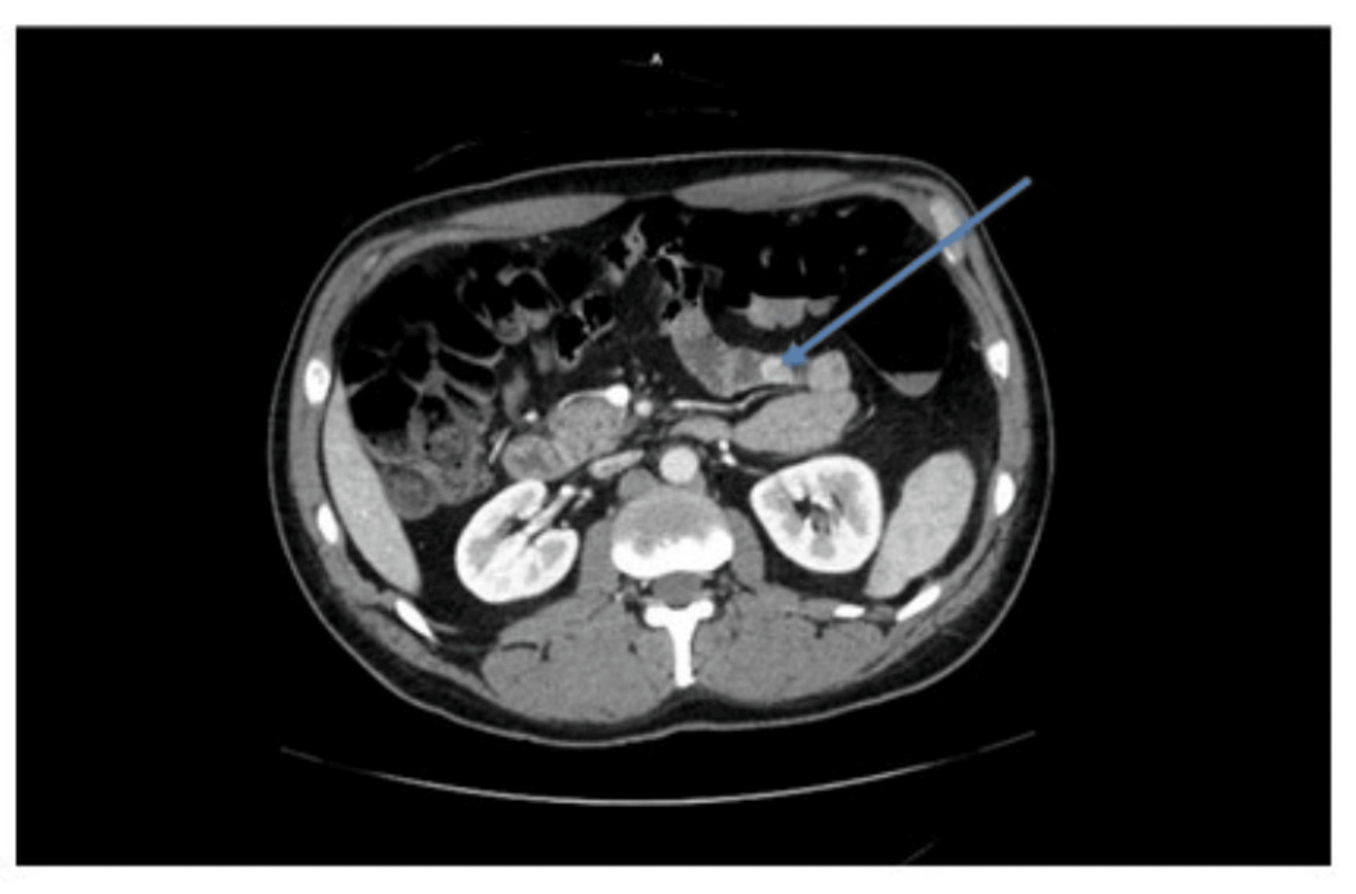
Hyperenhancement of the gastrointestinal stromal tumor within the small bowel on a CT scan with IV contrast
